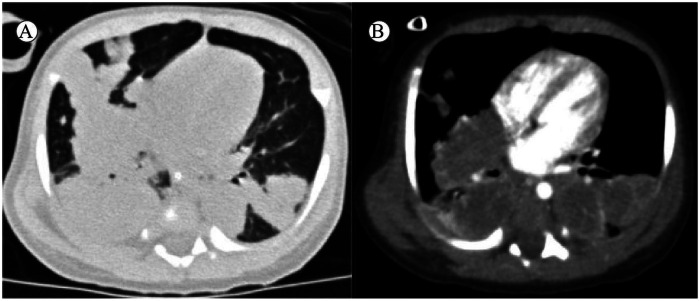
(A) chest CT scans demonstrate multiple patchy, large consolidations and mass opacities in both lungs. (B) Contrast-enhanced imaging reveals prominent, non-enhancing, hypodense necrotic changes within the lesions.
